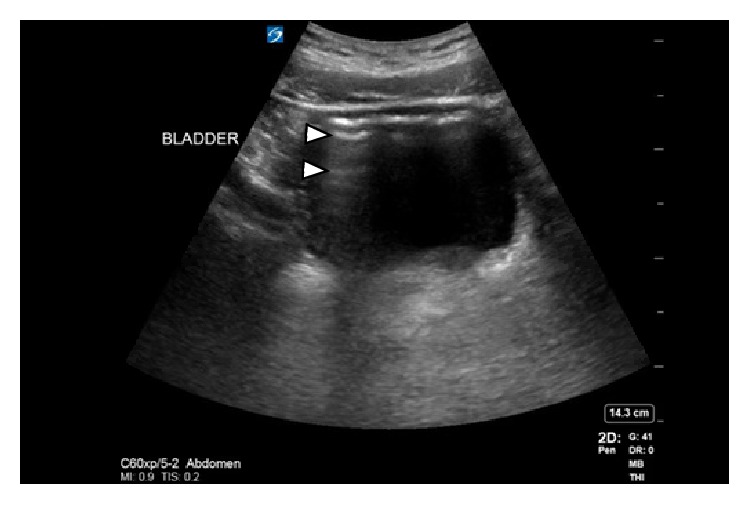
Transverse ultrasound of the bladder showing reverberation artifacts (triangles) in the dirty shadow cast by the tissue–gas interface in the urinary bladder.
